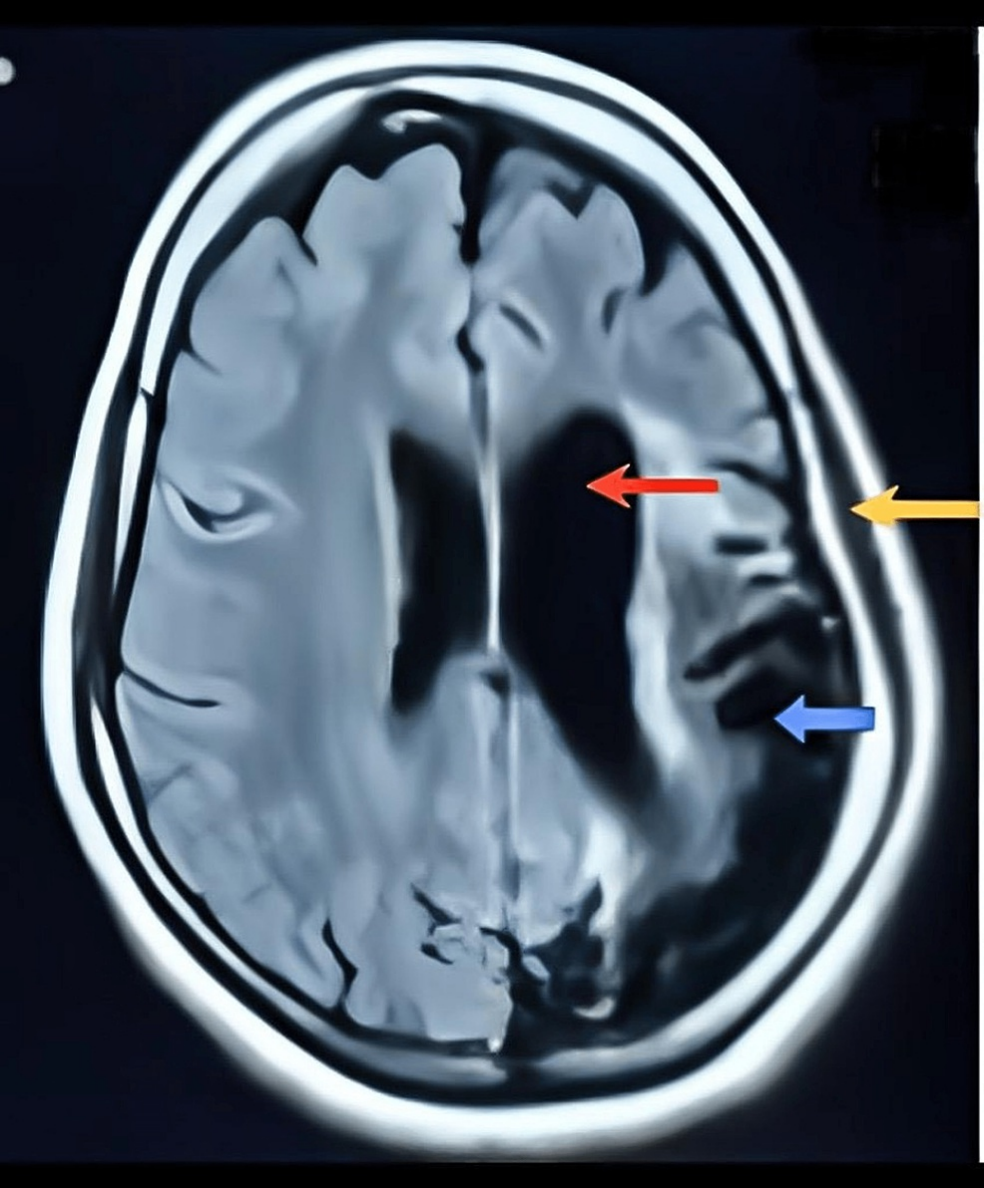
MRI brain scans of the patient. The red arrow shows ventricular dilatation of the left lateral ventricle. The blue arrow shows gliotic changes in the left temporoparietal and occipital lobes. The yellow arrow shows calvarial diploic space widening. MRI, magnetic resonance imaging
